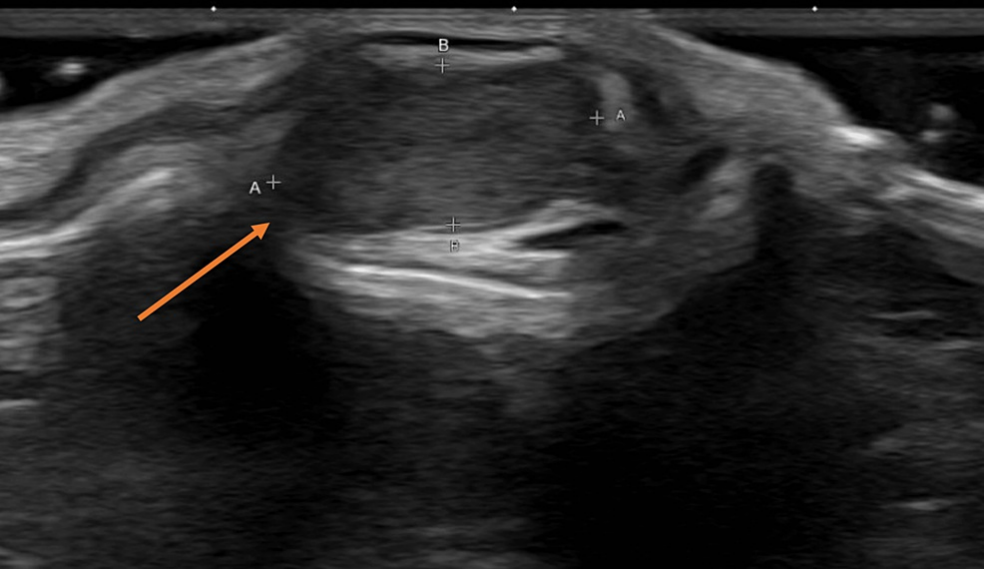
Ultrasound examination of the cyst on the left auricular cyst showing homogeneous, well-circumscribed, hypoechogenic mass (arrow).
